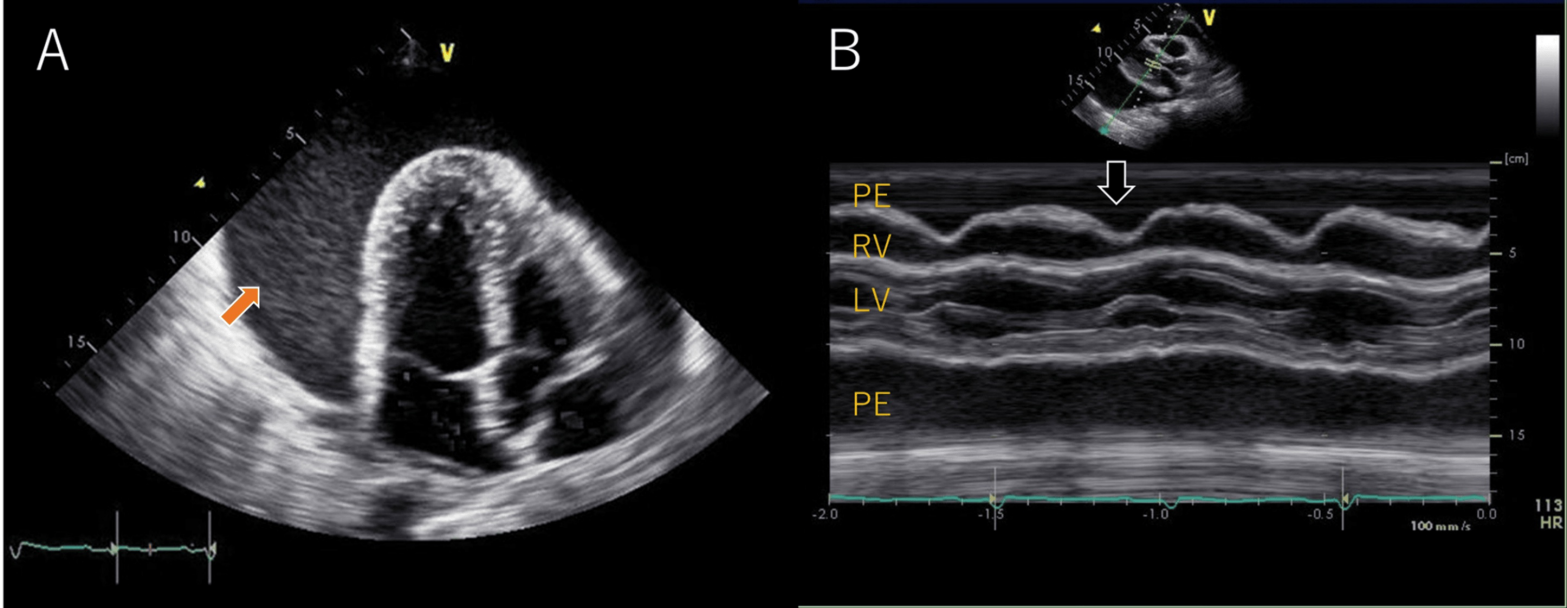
Echocardiography on admission A: Apical four-chamber view echocardiogram demonstrating features characteristic of cardiac tamponade (orange arrow). B: M-mode of diastolic right ventricular collapse in the parasternal long-axis view. Black arrows indicate the early diastolic collapse of the right atrium. PE: pericardial effusion, LV: left ventricle, RV: right ventricle
